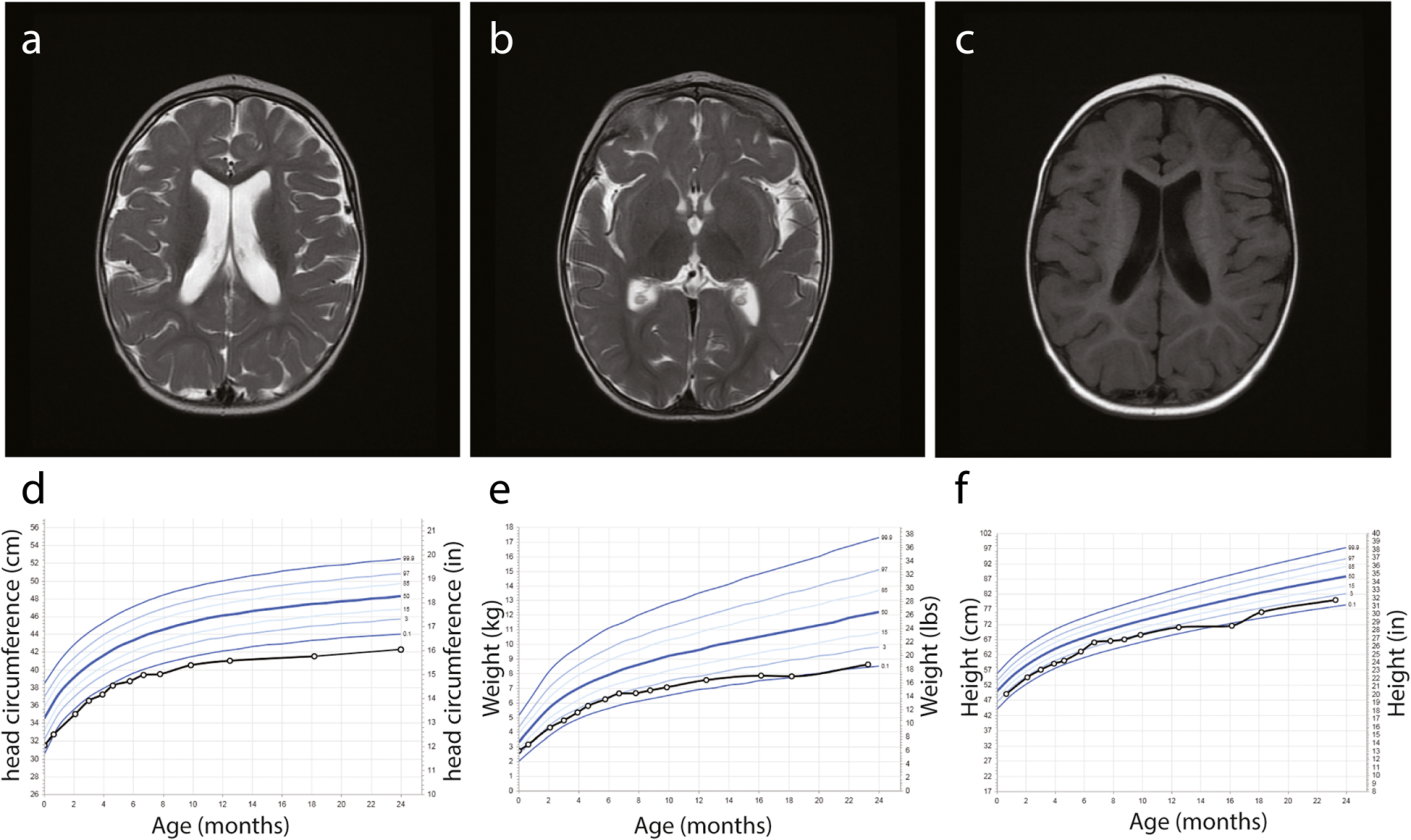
Clinical phenotype of the patient with *TTI2* mutation. a-b axial T2 images and c axial Flair image of brain magnetic resonance imaging (MRI) from the boy at 1 year of age. Small supratentorial ventriculomegaly and minor mixed diffuse cortical atrophy can be observed. d-f Growth curves for head circumference (d), weight (e) and height (f) from birth to the age of two years old. Blue line indicate percentile from age and sex matched controls
